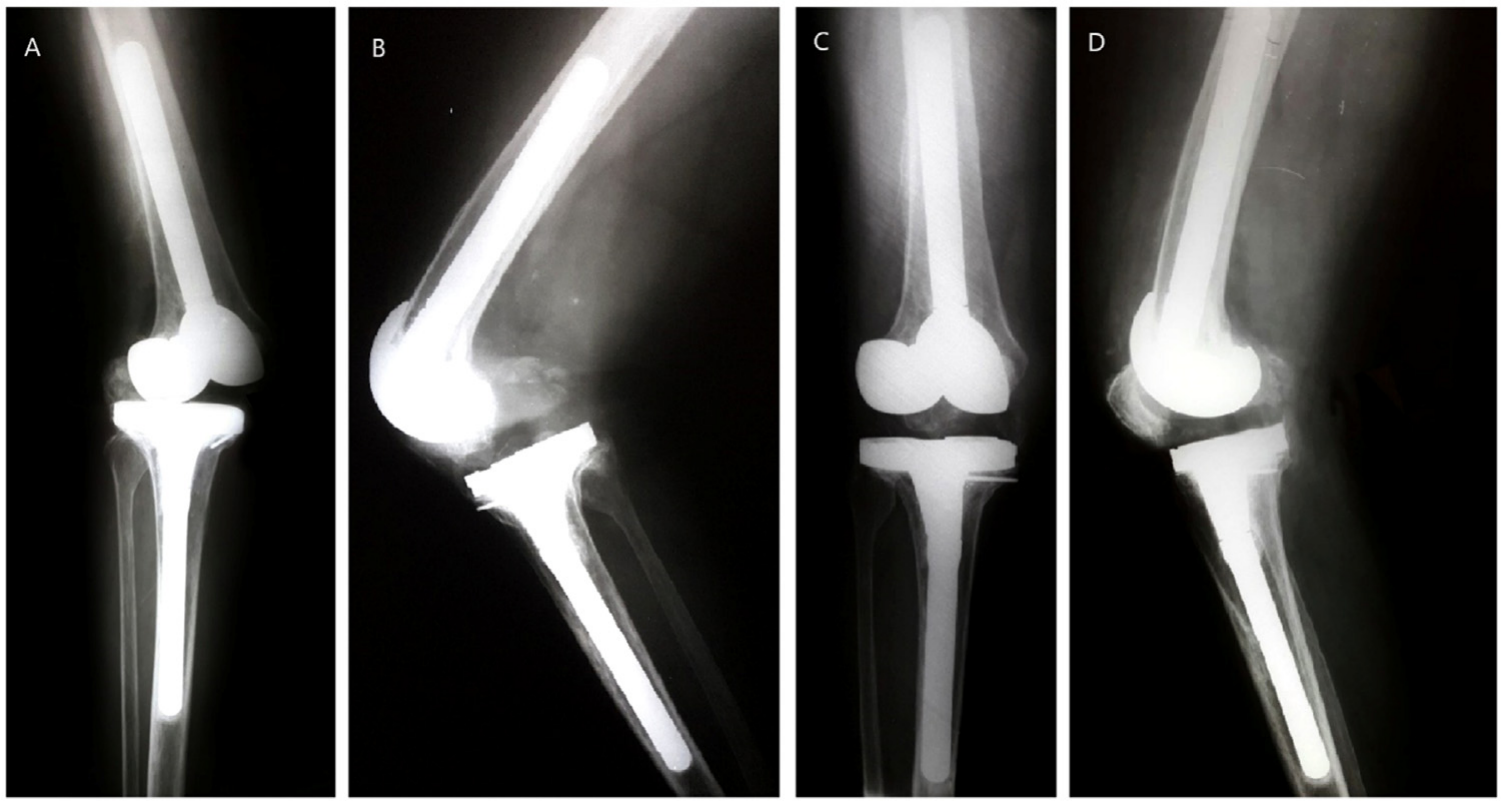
(A) Posterior dislocation. (B) Medial opening with avulsion of medial collateral ligament. (C) 6.1 years postoperative single leg standing AP X-Ray showing no medial opening. (D) Lateral view showing good position with patella baja.
